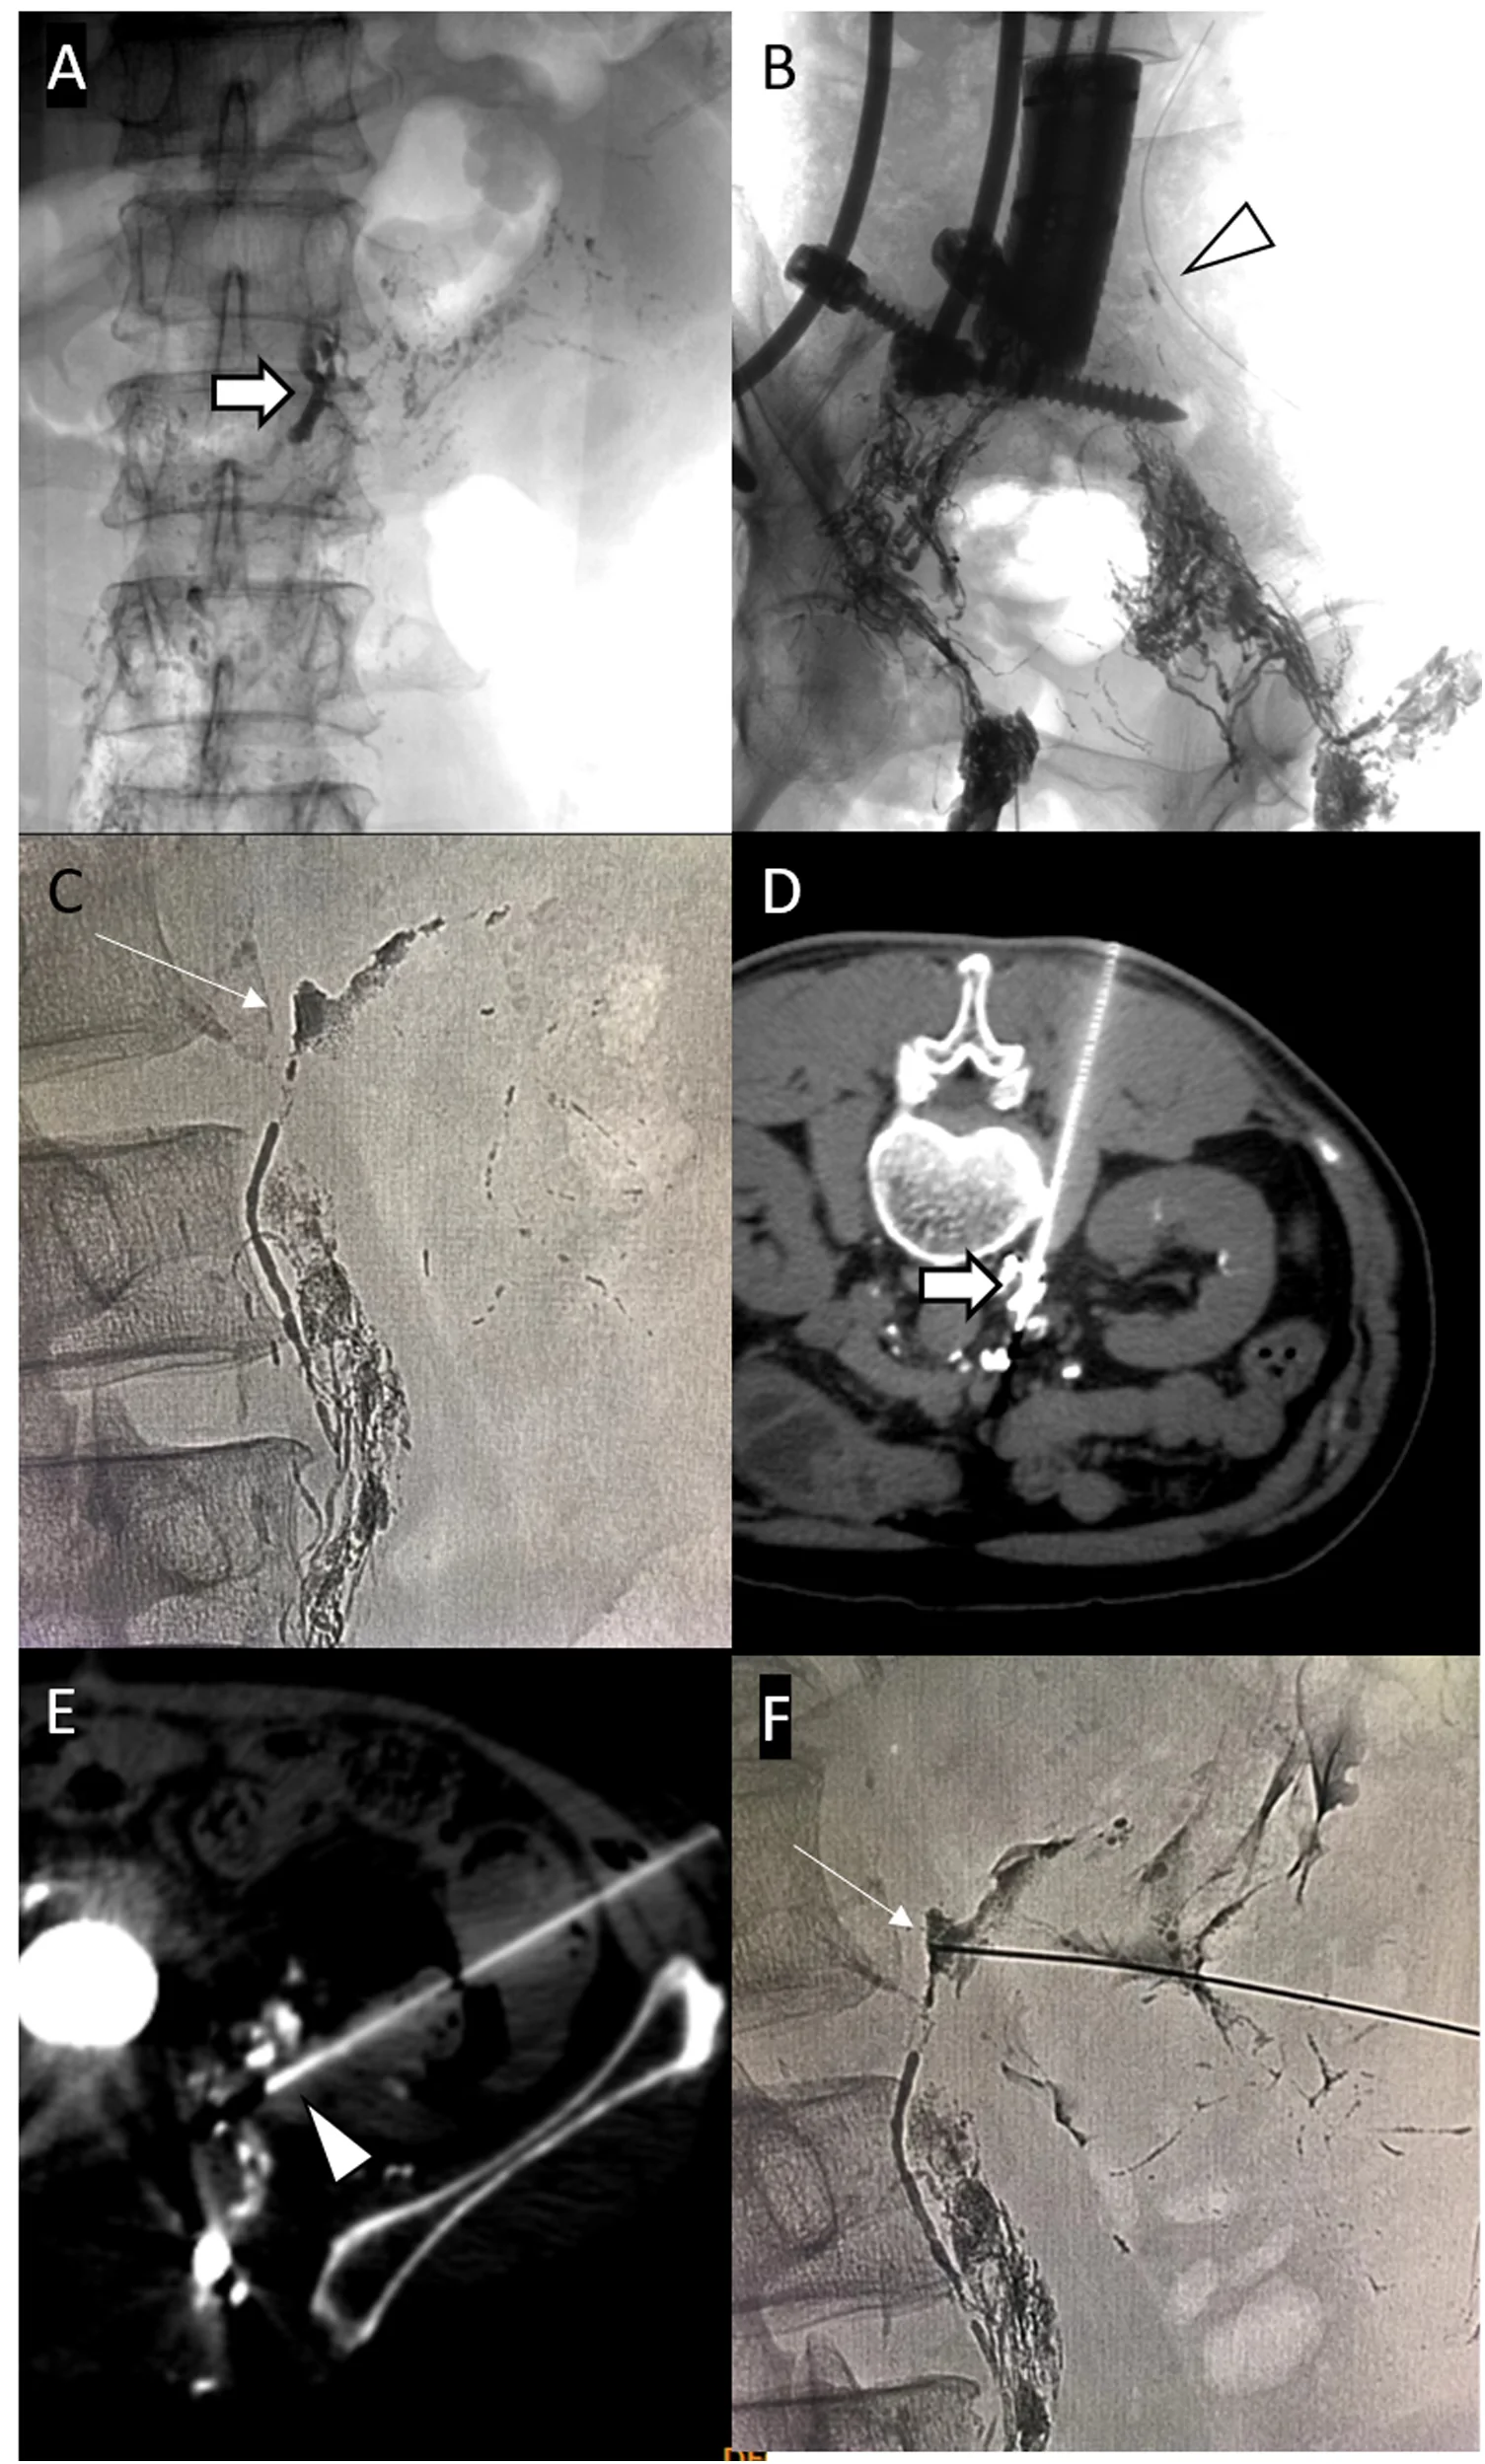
Typical interventional treatment images of lymphatic leakage. (A) A patient presenting with chyluria. Lipiodol lymphangiography demonstrates retrograde filling of Lipiodol into the left renal lymphatic system (arrow), confirming abnormal lymphatic-urinary communication. (B) A patient with chylous ascites following radical hysterectomy. Lipiodol lymphangiography reveals Lipiodol extravasation from the presacral lymphatic plexus (triangle arrow) into the peritoneal cavity, with subsequent accumulation within an abdominal drainage catheter (asterisk). (C) A patient with chylous ascites post-Whipple procedure (pancreaticoduodenectomy). Lipiodol lymphangiography identifies active leakage at the left L3 lumbar lymphatic trunk (thin arrow), with Lipiodol pooling in the peritoneal cavity. (D) CT-guided embolization of lymphatic vessels draining into the left renal region for (A). A mixture of Lipiodol and temperature-sensitive gel (TempSLE) was injected to occlude the pathological lymphatic channels (arrow), achieving complete resolution of chyluria. (E) CT-guided direct puncture of the lymphatic leakage site adjacent to the drainage catheter. TempSLE was injected to achieve targeted embolization (triangle arrow) for (B), resulting in immediate cessation of chylous fluid output. (F) Fluoroscopy-guided direct puncture of the leaking lumbar lymphatic vessel. A combination of TempSLE and Lipiodol was administered to embolize the leakage site (thin arrow), effectively sealing the defect and resolving ascites for (C).
